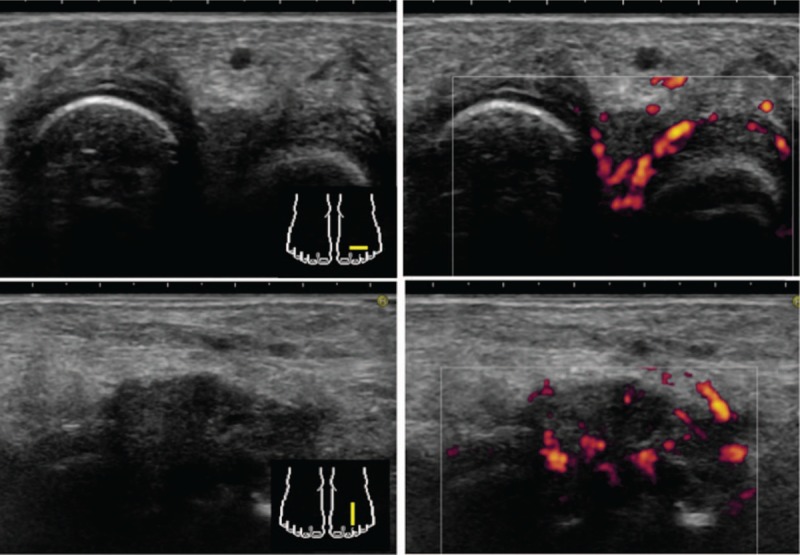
Cross and longitudinal section of the MSUS showed intermetatarsal bursitis with remarkable PD signals in the 2rd to 3th toes of left feet with mild synovitis in the left 3th MTP joint. MSUS = musculoskeletal ultrasound, MTP = metatarsophalangeal, PD = power Doppler.
